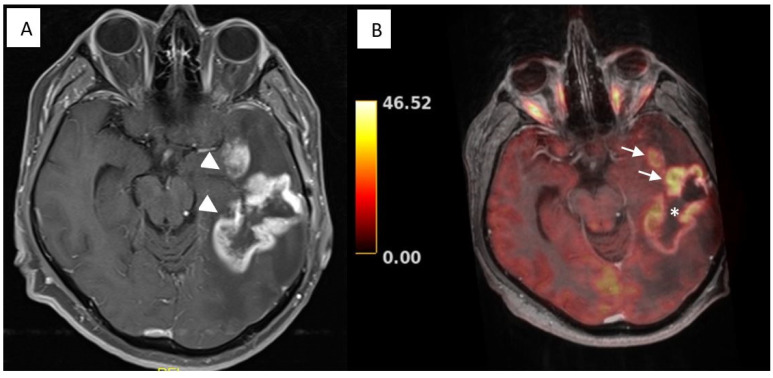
Case 5. T1-weighted magnetic resonance imaging (MRI) (A) revealed heterogenous contrasting lesions (arrowheads). Positron emission tomography/MRI images (B) showed focal hot spots (arrows) within the previously operated area (asterisk) as well as in a different area.
